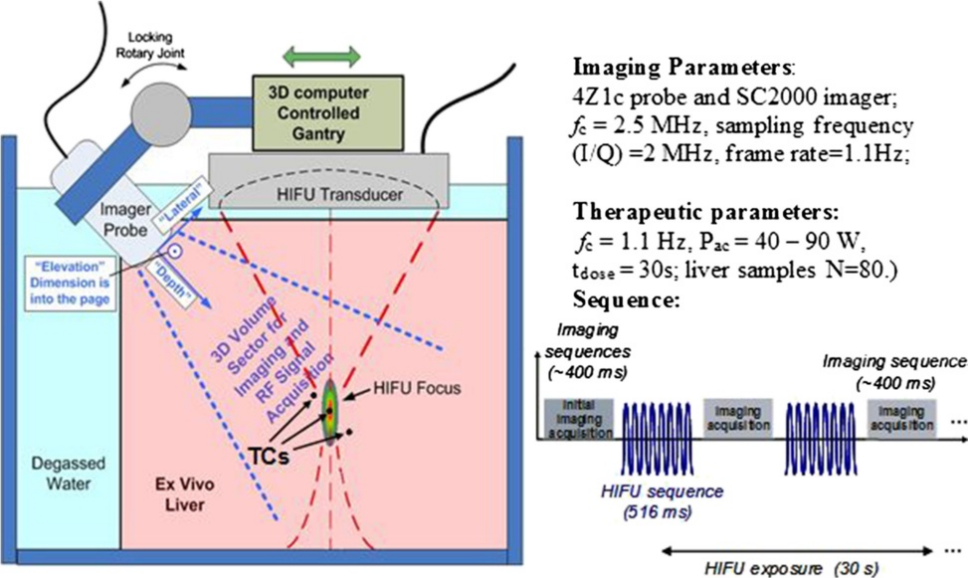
Acoustic thermometry experimental setup. Test fixture configuration for acquiring ultrasound echo data during HIFU heating experiments for thermometry data acquisition and RNN testing. Imaging and therapy interleave in sequence shown
